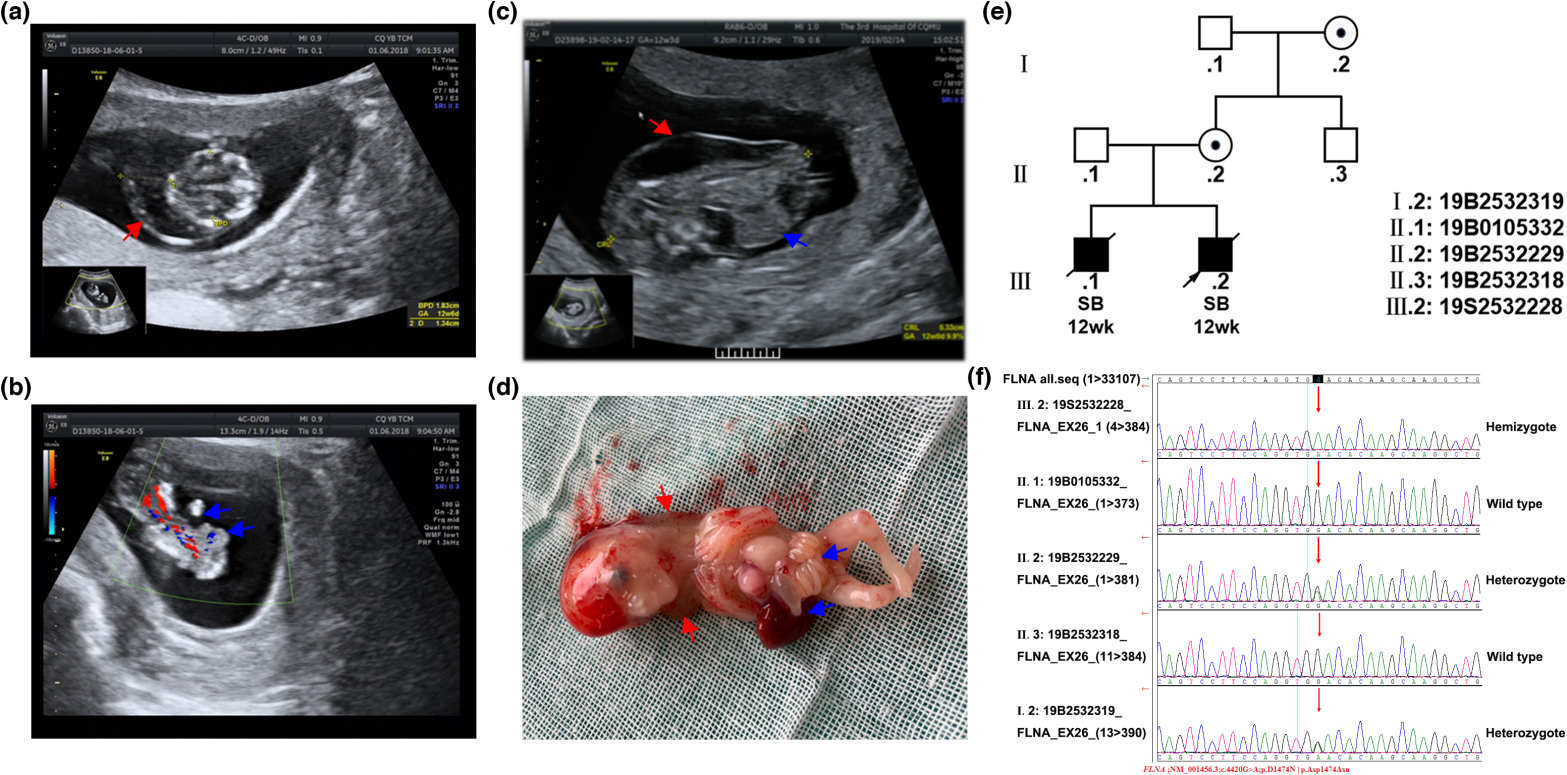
Ultrasonic examination and phenotype of the proband and his family genetic characteristics. (a, b) Ultrasonic examination of the first fetus (III.1) demonstrating hygroma colli (red arrow), omphalocele and unclear lower limbs (blue arrow). (c, d) Ultrasonic examination and phenotype of the second fetus (III.2) demonstrating hygroma colli (red arrow), thoracoceloschisis with visceral valgus (blue arrow) and abnormal lower limbs. (e) The genetic spectrum for this family with *FLNA* mutation; SB means stillbirth. (f) The mutational locus of *FLNA* (c.4420G>A) in this family by WES.
